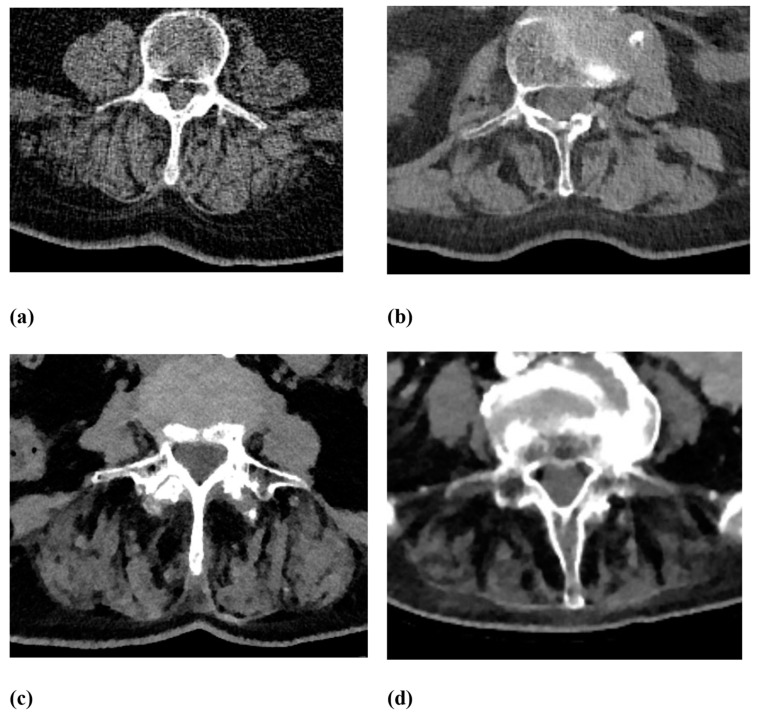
Goutallier score modified for CT scans along the lumbar spine. (a) Grade 1 for a few fatty streaks within the muscle. (b) Grade 2 for less fat than muscle within the muscle. (c) Grade 3 for the same amount of fat and muscle within the muscle. (d) Grade 4 for slightly fatter than muscle within the muscle.
